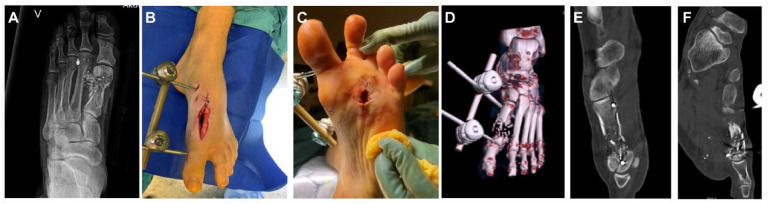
The figure shows the anteroposterior radiograph of the left foot demonstrating the projectile fragments and a shortened fragmented first metatarsal (1A), the left foot with a gunshot injury with a debrided wound dorso medial and central plantar (1B and C). The foot is stabilized with an external fixator, and computed tomography scans of the injury demonstrating the metatarsal defect and metatarso phalangeal joint (1D, E and F).
